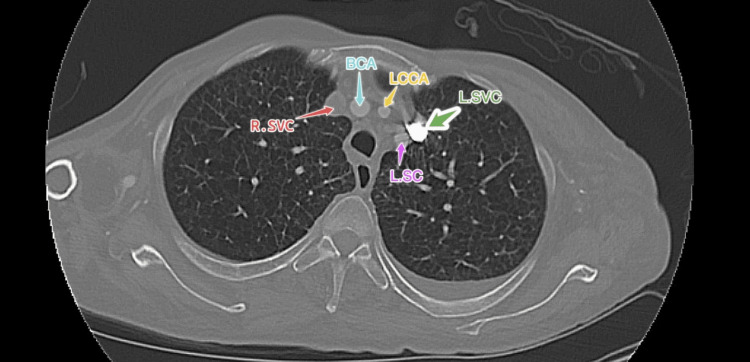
Computed tomography angiography scan demonstrating duplication of superior vena cave R.SVC - right superior vena cava; BCA - brachiocephalic artery; LCCA - left common carotid artery; LSC - left subclavian artery; L.SVC - left subclavian artery
